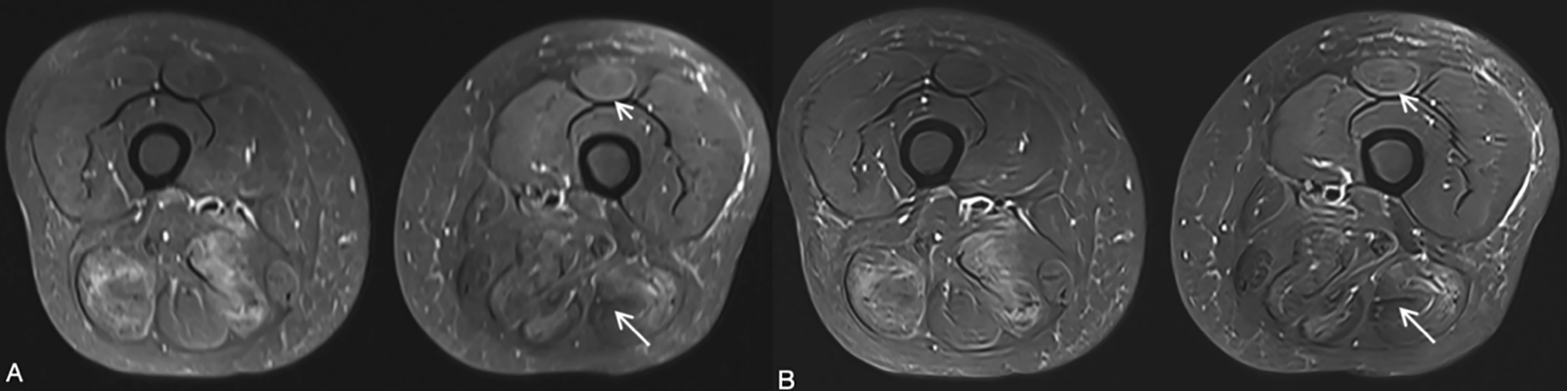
Thigh MRI images of a 48-year-old female patient with immune-mediated necrotizing myopathy. (A) Standard TSE. (B) TSE_DL_. The edge sharpness and contrast resolution of TSE_DL_ image is better than that of Standard TSE image. On TSE_DL_ image, the left biceps femoris tendon (long arrow) is nicely delineated, and fasciitis of the left rectus femoris (short arrow) is evident. By contrast, the left biceps femoris tendon (long arrow) and fasciitis of the left rectus femoris (short arrow) are vaguely shown on standard TSE image.
